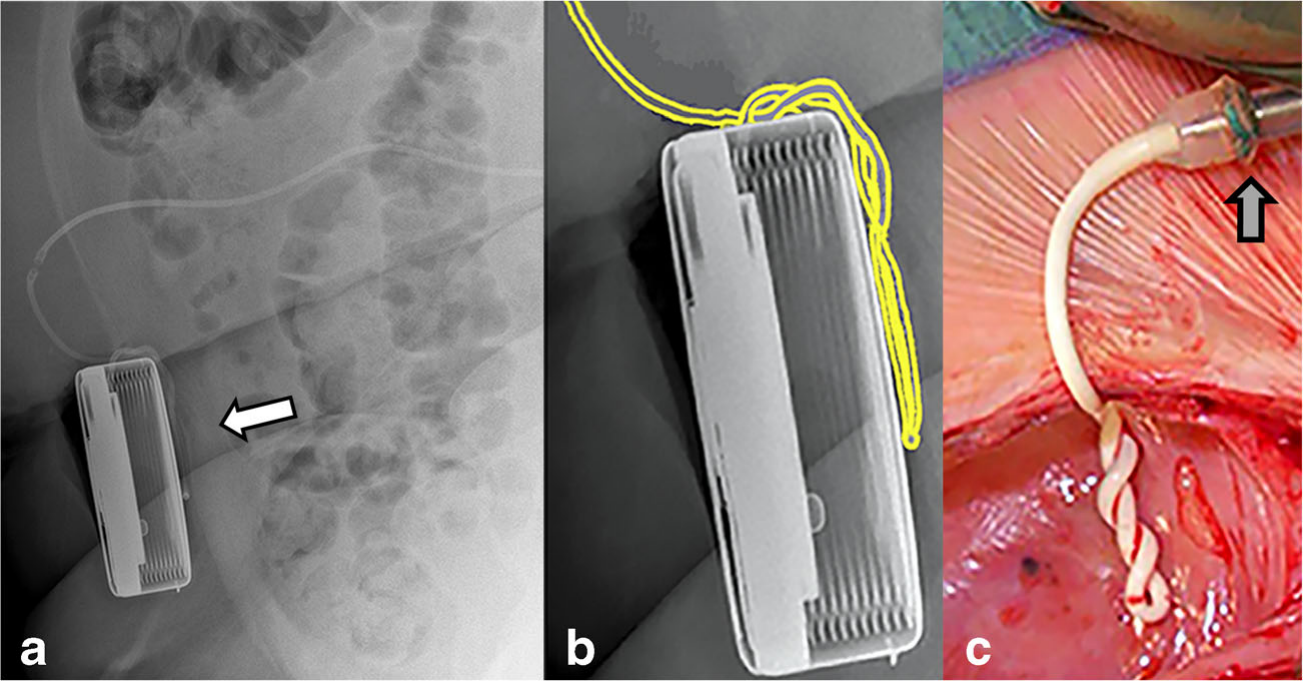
Twiddler’s syndrome with signs of withdrawal syndrome in a 51-year-old woman with dystonia in complex regional pain syndrome. A repeatedly twisted old type catheter is visible on lateral plain radiography behind the implanted pump (*white arrow*) (a), magnification of the pump with artist rendering (b) and an in vivo image (c). The catheter is fixed to the pump with an old sutured connector (*grey arrow)*
